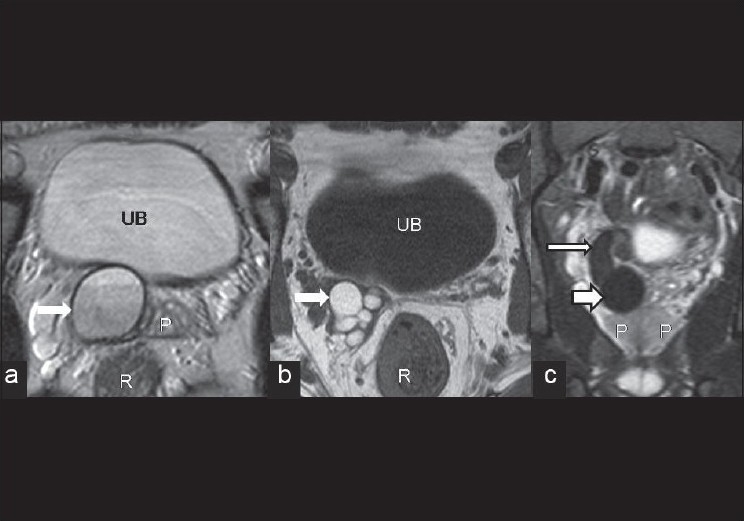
(a) Abdominal MRI T2-weighted axial image acquired using TSE sequence showing a large thick-walled cyst in right seminal vesicle with hyperintense contents and presence of fluid–fluid level within (arrow). (b) Abdominal MRI T1-weighted axial image acquired through a more cranial level showing hyperintense contents in the proximal part of dilated right seminal vesicle (arrow). (c) STIR coronal MRI showing continuity of cyst (short broad arrow) with the right seminal vesicle (long thin arrow). P, prostate; R, rectum; UB, urinary bladder
